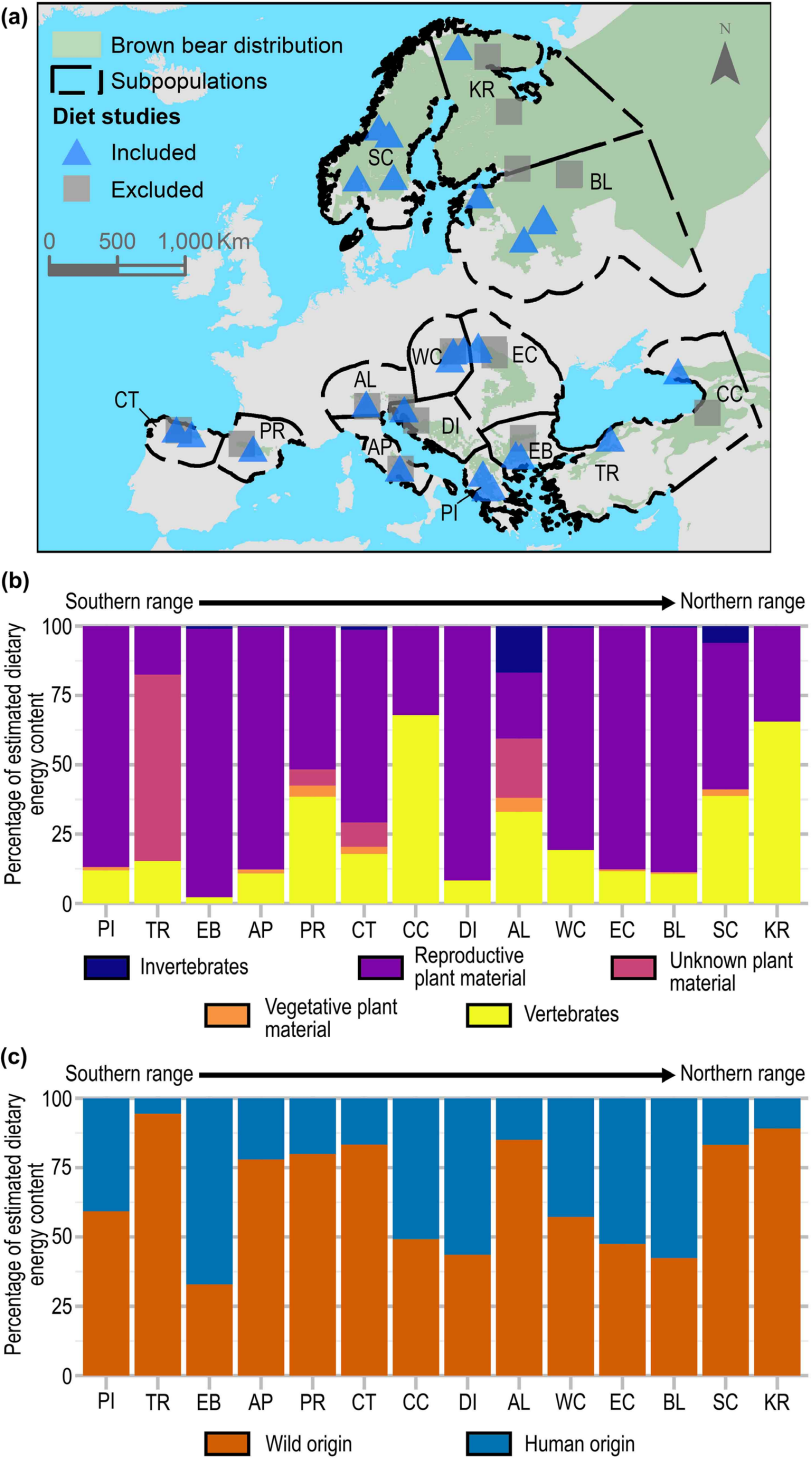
Brown bear diet in Europe. (a) Map showing the 14 brown bear subpopulations considered: Pindos (PI), Türkiye (TR), East Balkan (EB), Apennine (AP), Pyrenees (PR), Cantabrian (CT), Caucasian (CC), Dinaric (DI), Alpine (AL), Western Carpathian (WC), Eastern Carpathian (EC), Baltic (BL), Scandinavian (SC), and Karelian (KR). We also mark the location of all the studies of brown bear diet reviewed, indicating if they were ultimately included (*n* = 31) or not (*n* = 16) for the calculation of biotic interactions at species level and for the associations between diet and environmental variables; note that not all studies are visible due to the overlapping of locations. (b) Relative estimated dietary energy content (rEDEC, a proxy for the relative importance of each item in the diet) identified at the species level for each food category, for each of the 14 brown bear subpopulations in Europe. (c) Proportion of the rEDEC for wild species and those of human origin.
